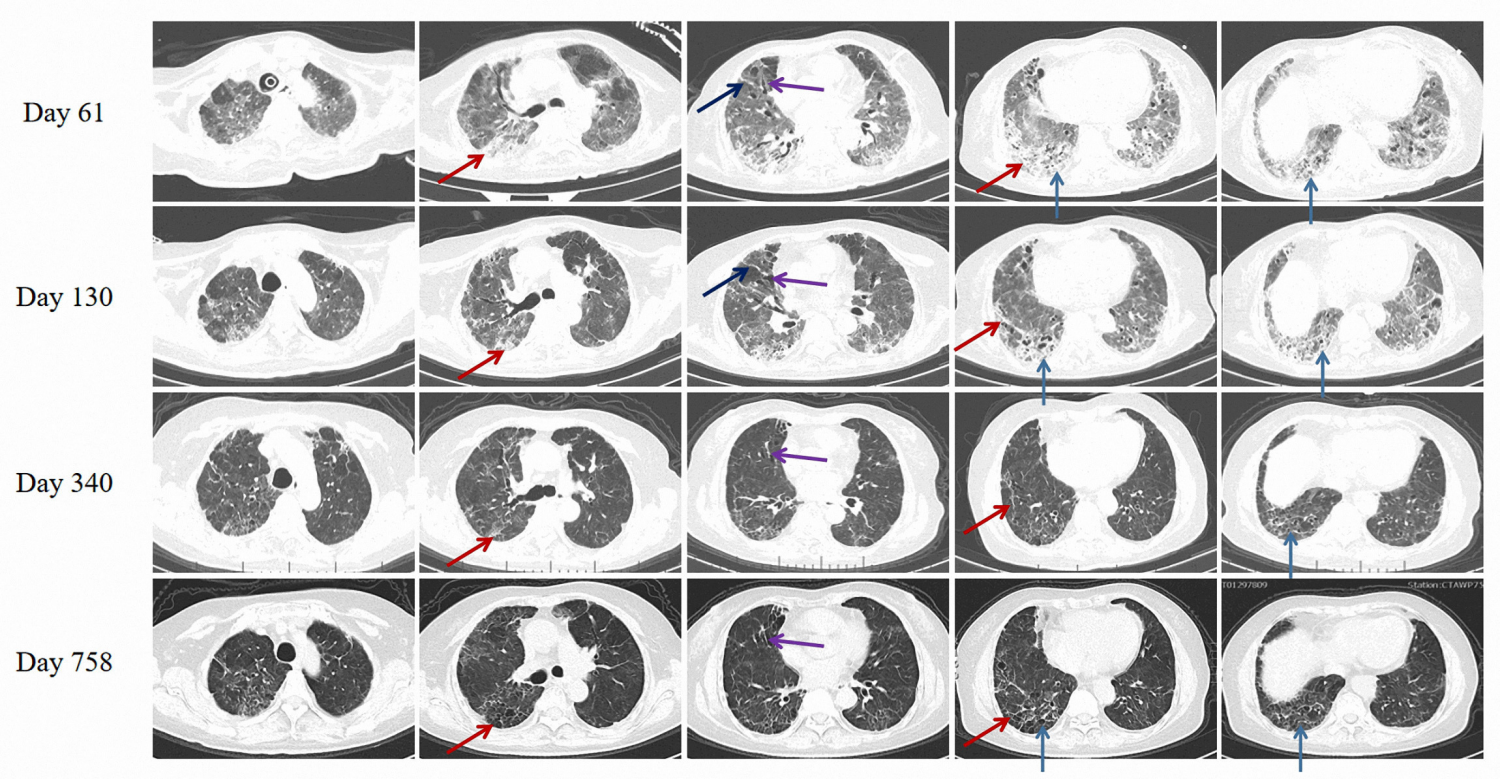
Chest CT on day 61 (Starting taking pirfenidone), day 130 (At discharge), day 340 and day 758 after illness onset. Red solid arrows: subpleural reticular abnormalities and fibrous cords. Black solid arrow: interlobular septal thickening. Purple solid arrow: traction bronchiectasis. Blue solid arrow: honeycomb-like change under the subpleura.
